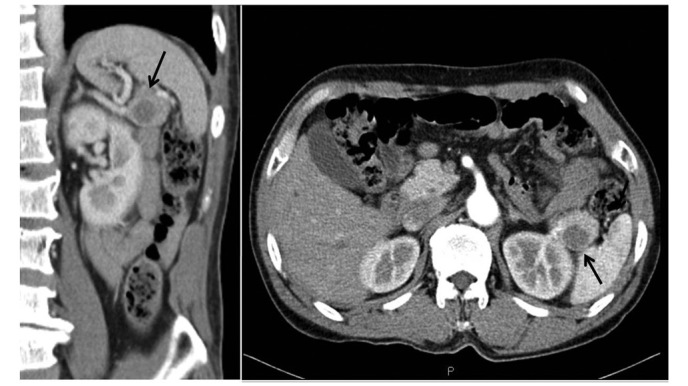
Radiological examination. Enhanced abdominal computed tomography (CT) reveals a clearly defined, 2.3 cm-sized nodule in the pancreatic tail (arrow).
